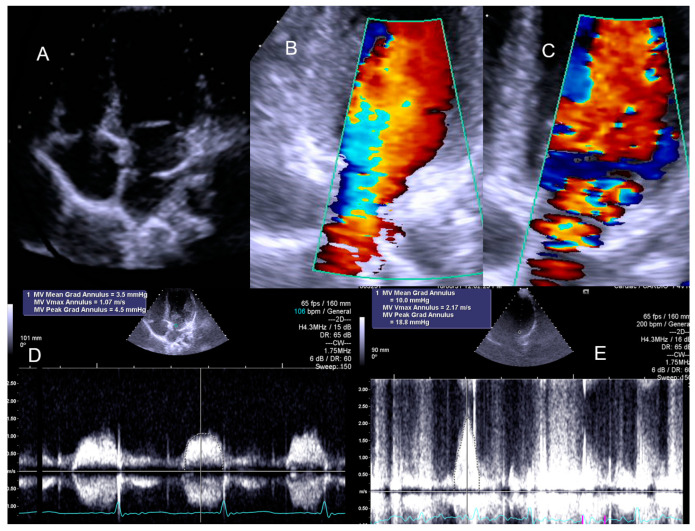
The exercise Doppler data in conjunction with the exercise and clinical data led the medical team to keep the patient in close clinical follow-up. (A): Intra–atrial septum in “cortriatriatum”; (B): color flow before exercise; (C): color flow at peak exercise; (D): continuous–wave (CW) flow before exercise; (E): CW flow at peak exercise [1].
